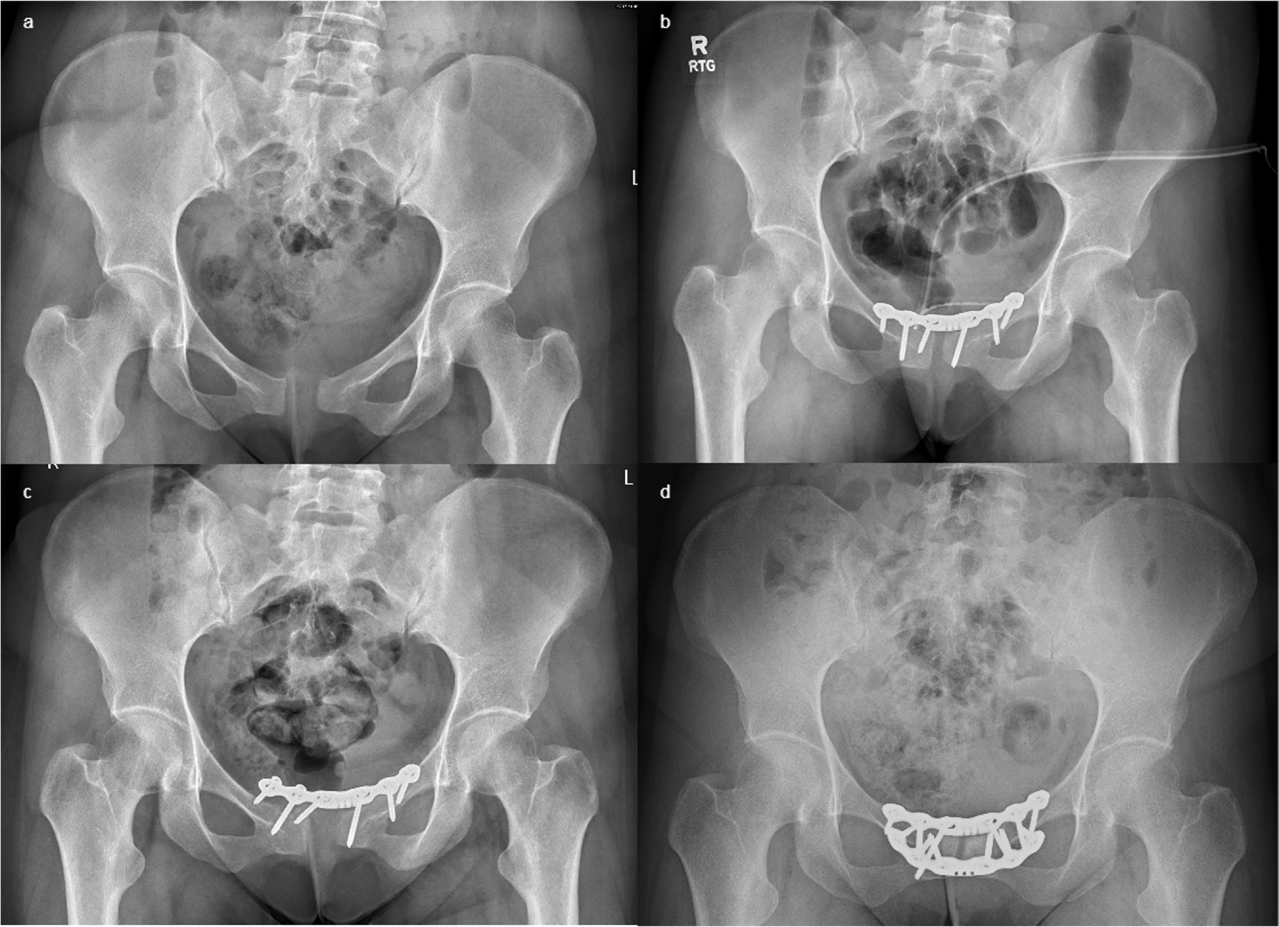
Case 4.A 30-year-old woman, who had given birth 6 months prior, complained of pain and a sensation of instability in her pelvis. a Initial plain radiograph shows a 20 mm wide and 6 mm vertical diastasis of the pubic symphysis. b The immediate postoperative film after open reduction and internal fixation of the pubic symphysis with a 6-hole plate. c Three-months follow-up radiograph showing plate failure. d Five-years follow-up radiograph after revision surgery, re-reduction and fixation with two orthogonal 6-hole plates. The pubic symphysis remains reduced
